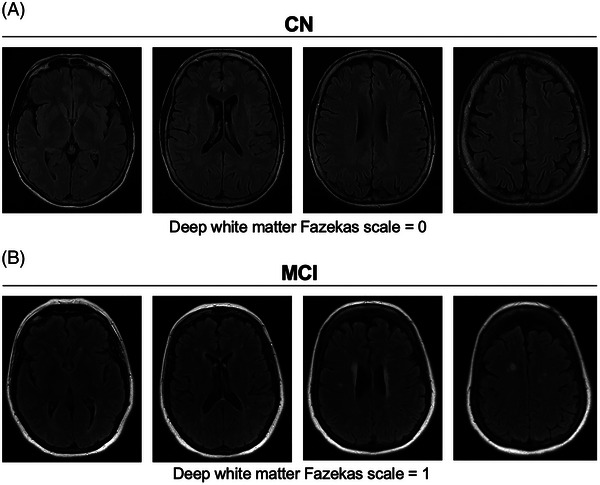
Clinical grading of small vessel ischemic lesions in control and participants with mild cognitive impairment (MCI). (A) Control (CN) participant (age: 68 years old, sex: female) with historical magnetic resonance imaging (MRI), recorded in 2023 shows a Fazekas scale grading of 0, indicating no small vessel ischemic damage determined by this grading classification. (B) Participant with MCI (age: 65 years old; sex: female) historical MRI, recorded in 2023, shows a Fazekas scale grading of 1, indicating moderate small vessel ischemic damage. Fazekas scale grading was assessed for deep white matter hyperintensities.
